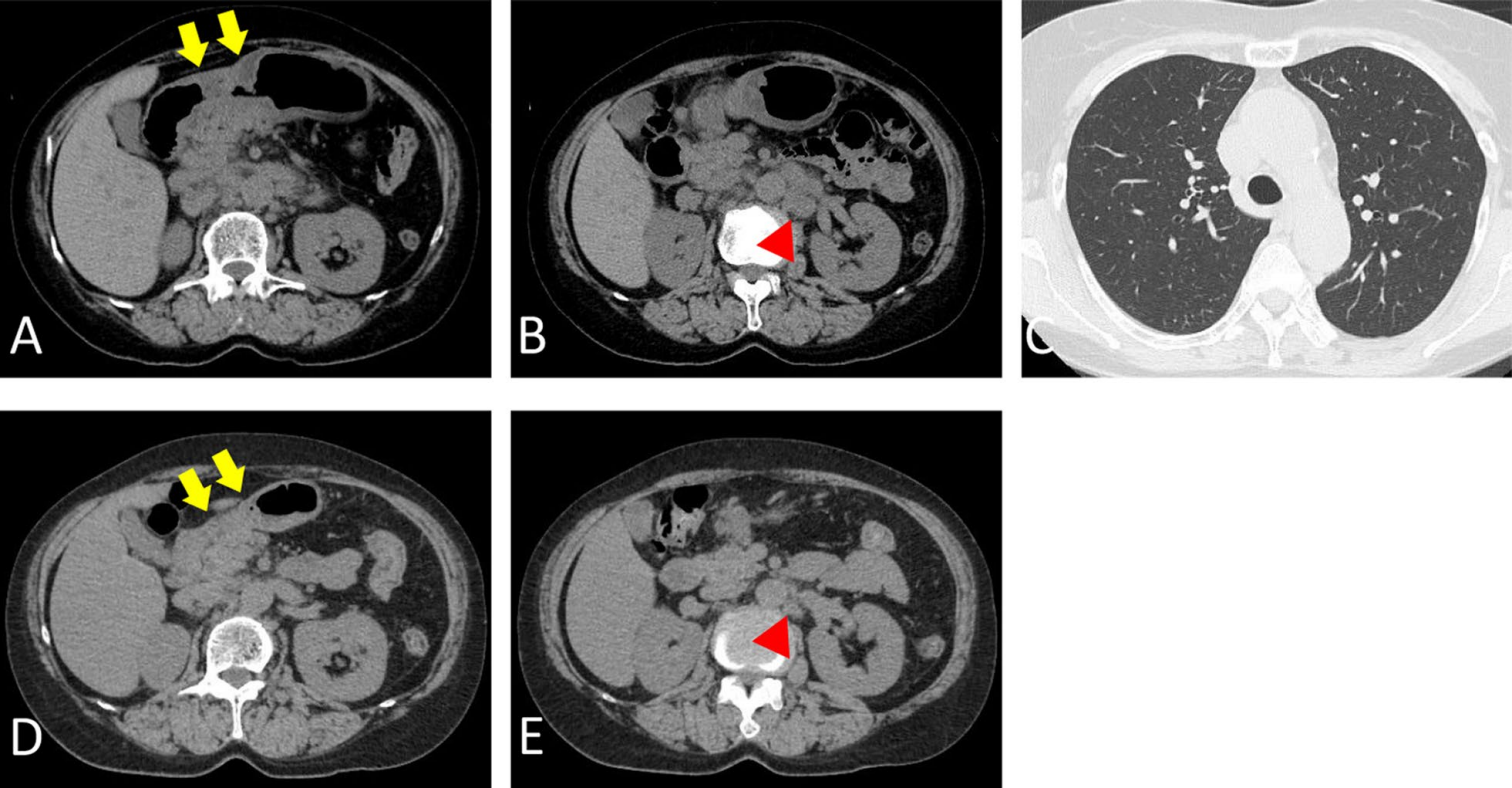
CT in Case 2. Arrows, primary gastric tumor; arrowheads, node metastases around abdominal aorta. A Before starting chemotherapy, CT shows a mass formed by the tumor of the gastric antrum. B CT shows multiple node metastases around the abdominal aorta. C CT of the lungs revealed no obvious lesions. D After 3 cycles of chemotherapy, CT shows shrinkage of the primary tumor. E CT shows shrinkage of the node metastases around the abdominal aorta
